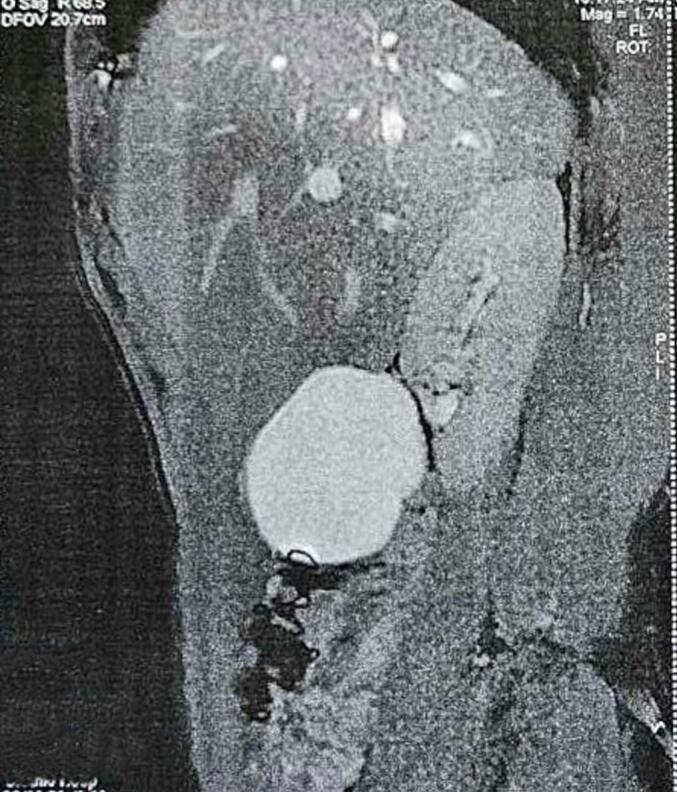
MRI images of the peritoneal cyst.
